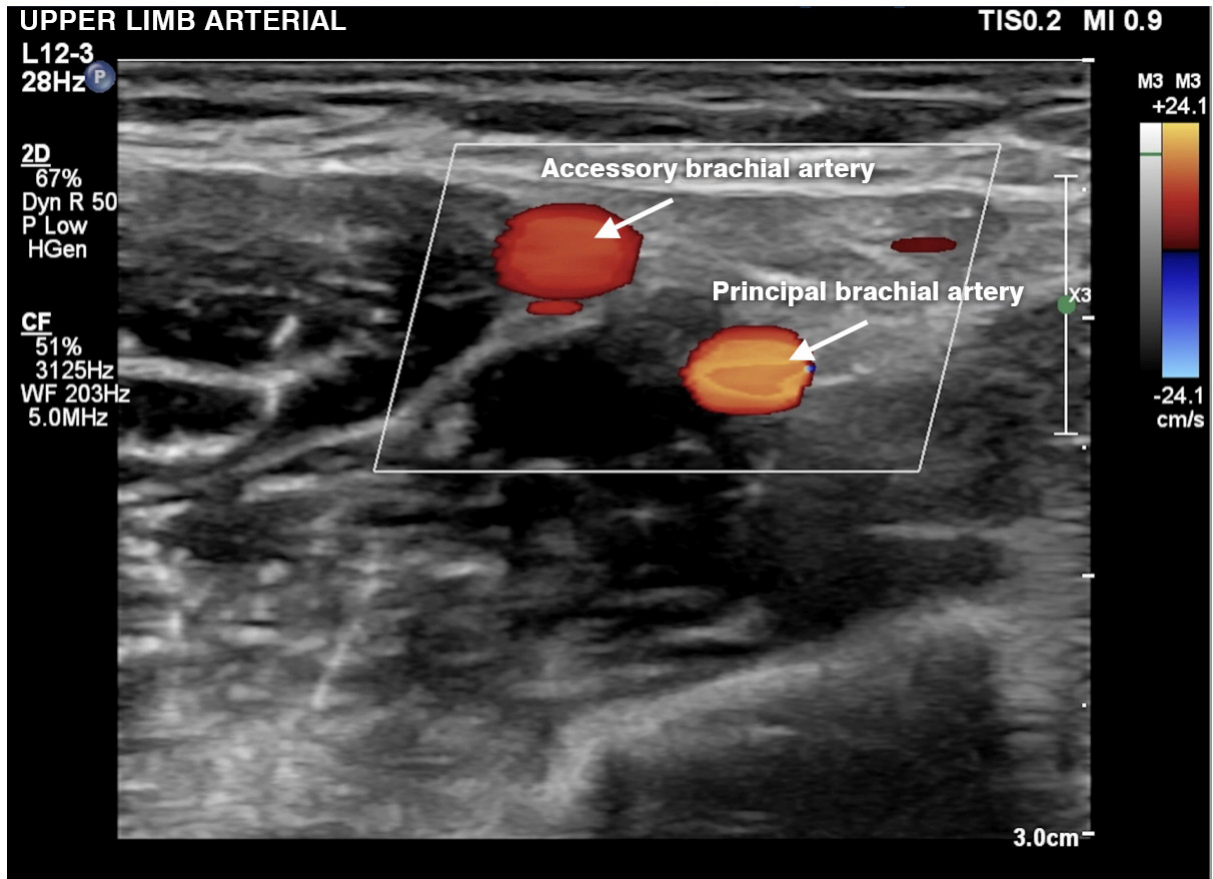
Doppler ultrasound in transverse view showing an accessory brachial artery and the principal brachial artery, indicating that there is an anatomic variant of the artery.
